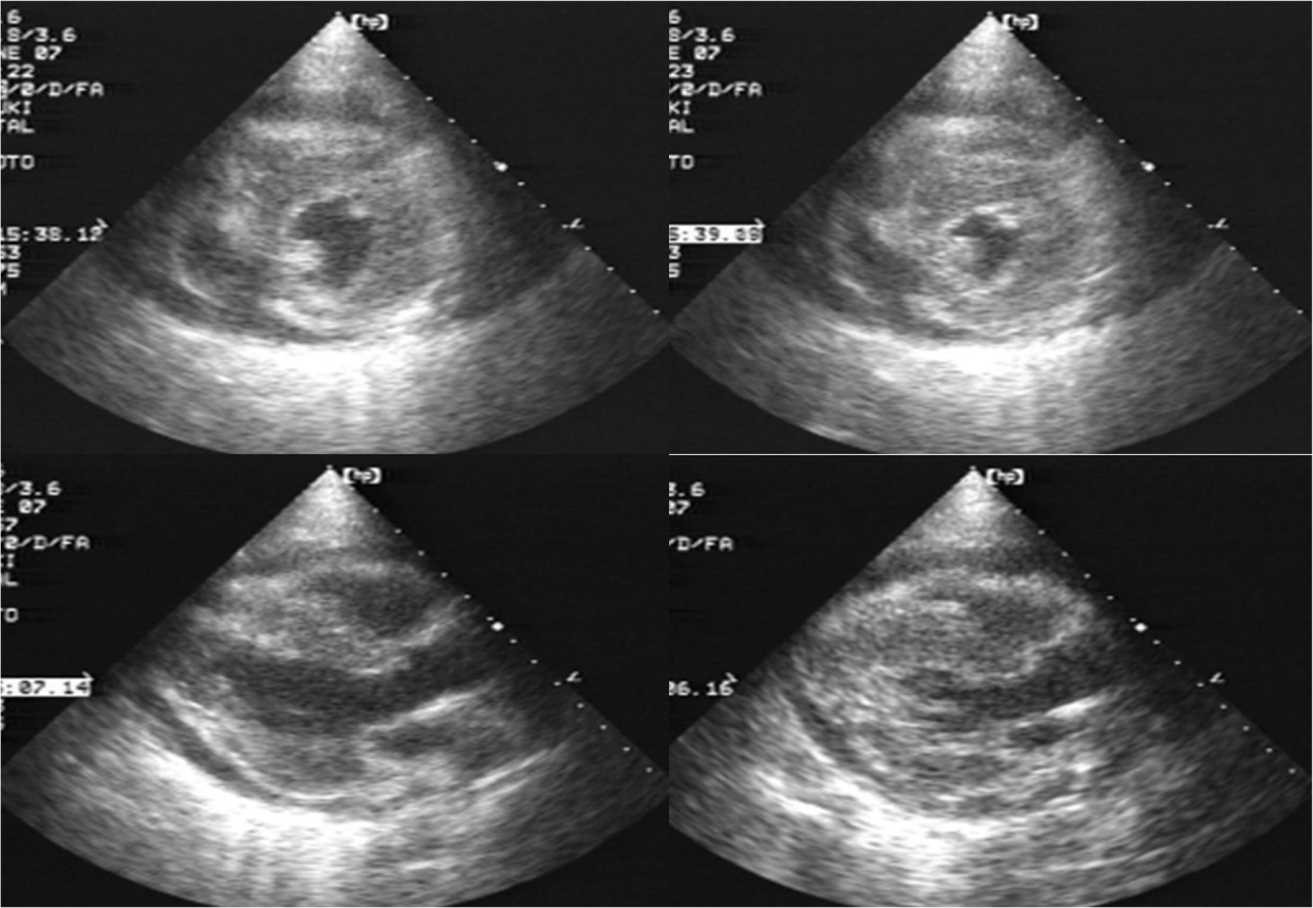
Echocardiogram on admission. Long axial view (left) and short axial view (right) obtained via a parathoracic approach. Almost full-circumferential thickening of the left ventricular wall and massive pericardial effusion are noted.
